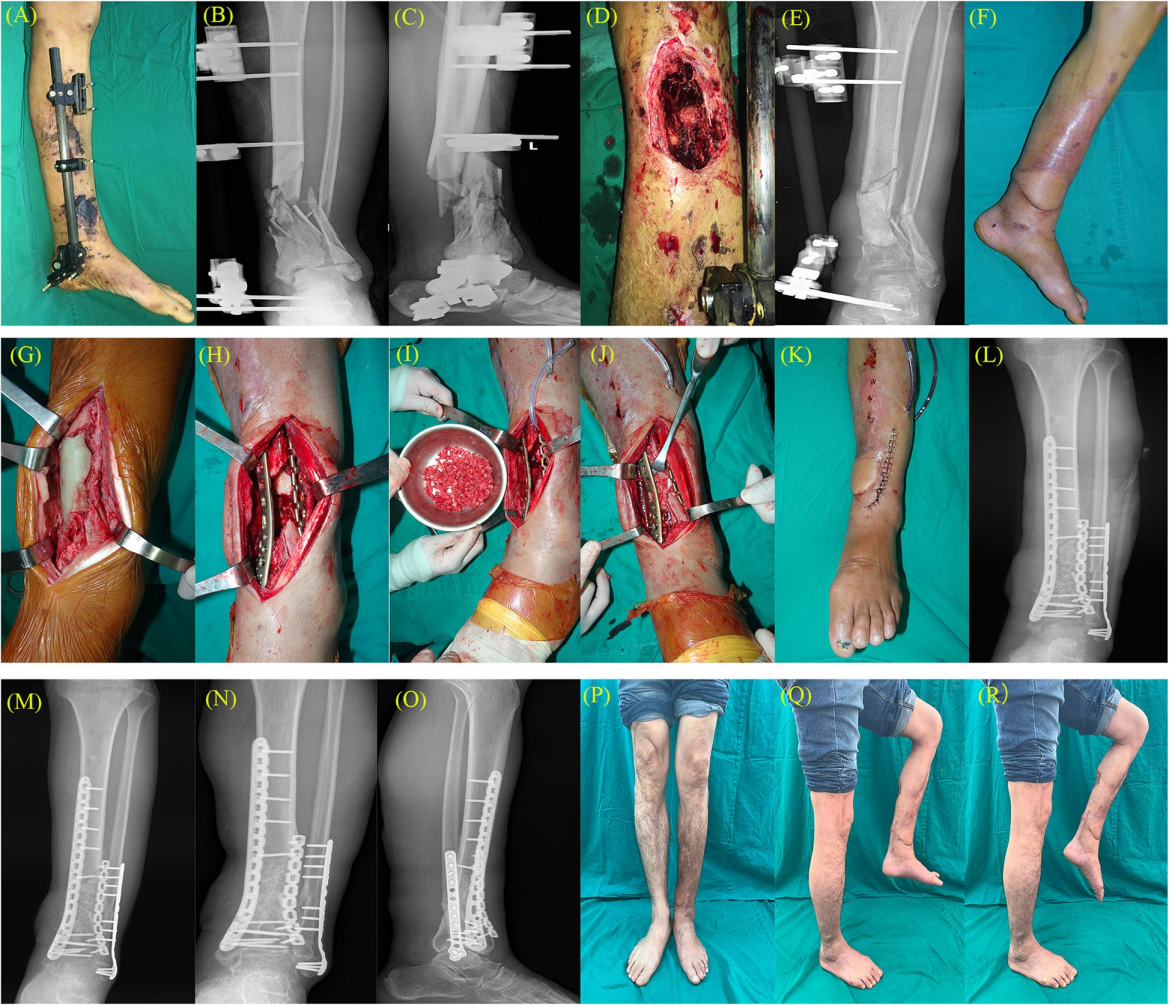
A/B/C/D The patient was transferred from an external hospital after being injured. The left medial leg was infected, the necrotic soft tissue and infected bone were cleared, and Staphylococcus aureus was detected. E/F The ipsilateral peroneal artery perforator flap was used to reconstruct soft tissue defect. G/H/I/J/K The induced membrane was cut longitudinally and the cement spacer was removed. The distal tibia was fixed with double plates, and iliac bone was removed to make bone granules, which were packed into the defective tibial region. L Postoperative X-ray after bone grafting. M Follow up X-ray one year after bone grafting surgery. N/O Follow up X-ray after bone grafting for 2 years. P/Q/R After 26 months of surgery, the patient's left calf recovered well.The Iowa ankle score was 86
